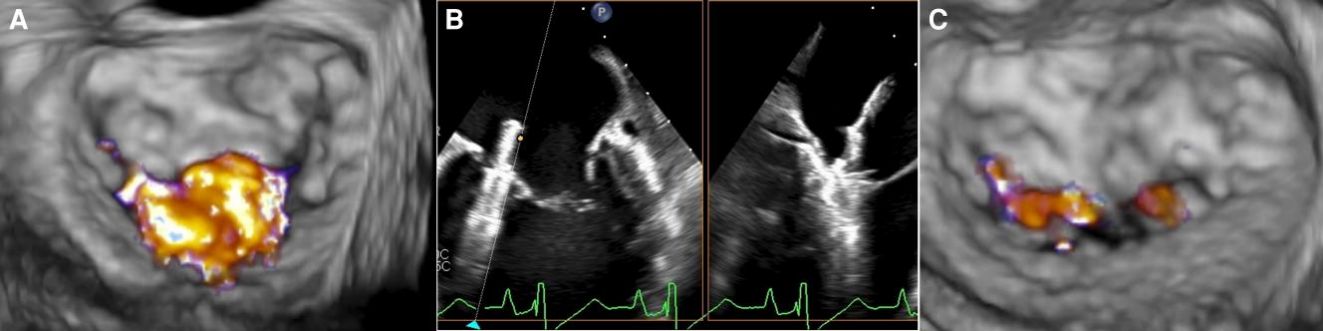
TEE images before and after the first procedure. Baseline 3D-TEE showing severe MR (*A*). XTW implantation for medial A2/P2 (*B*) and mild MR seen in postprocedural TEE (*C*). MR, mitral regurgitation; TEE, transoesophageal echocardiography.
